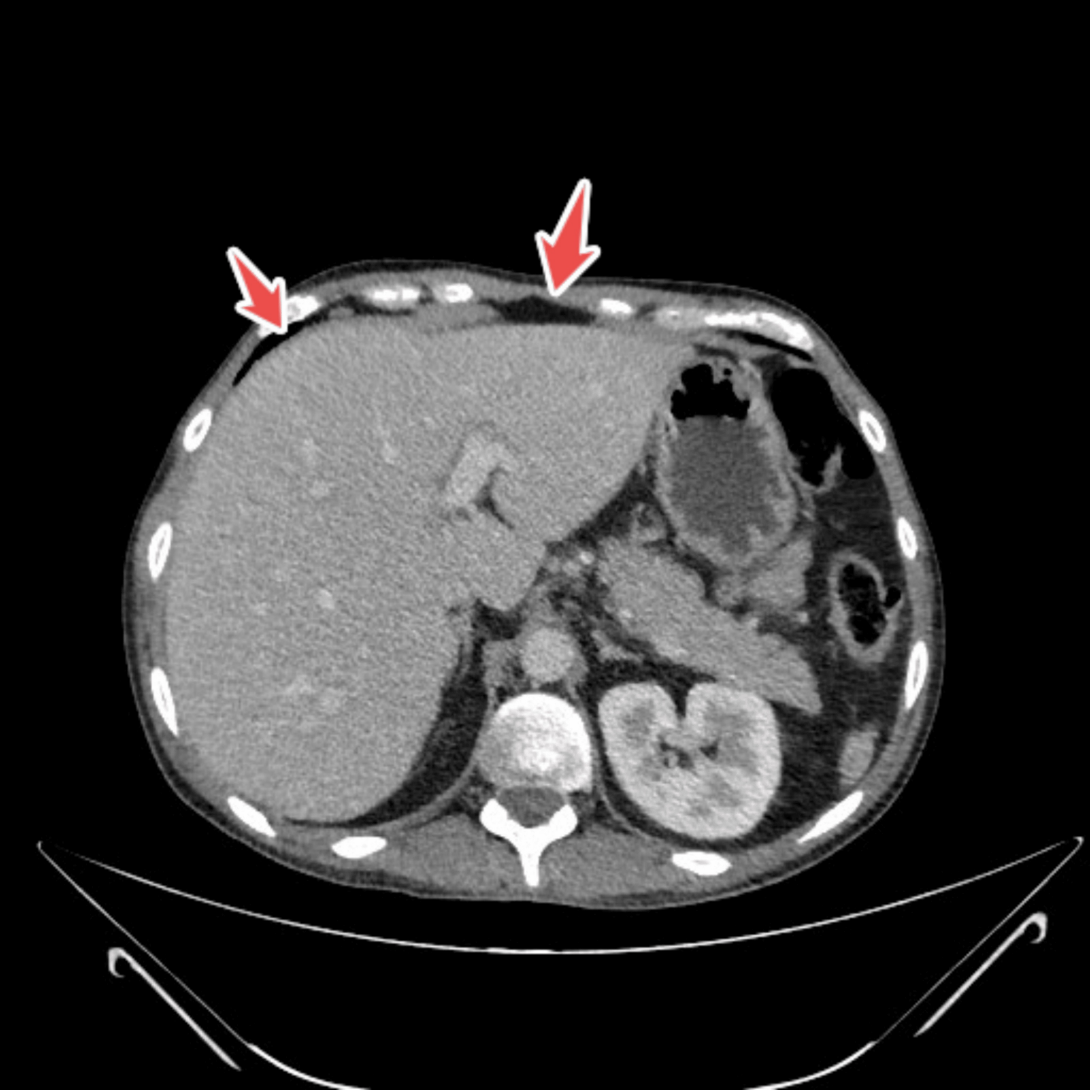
CT abdomino-pelvic showing pneumoperitoneum, highlighted by arrows.
